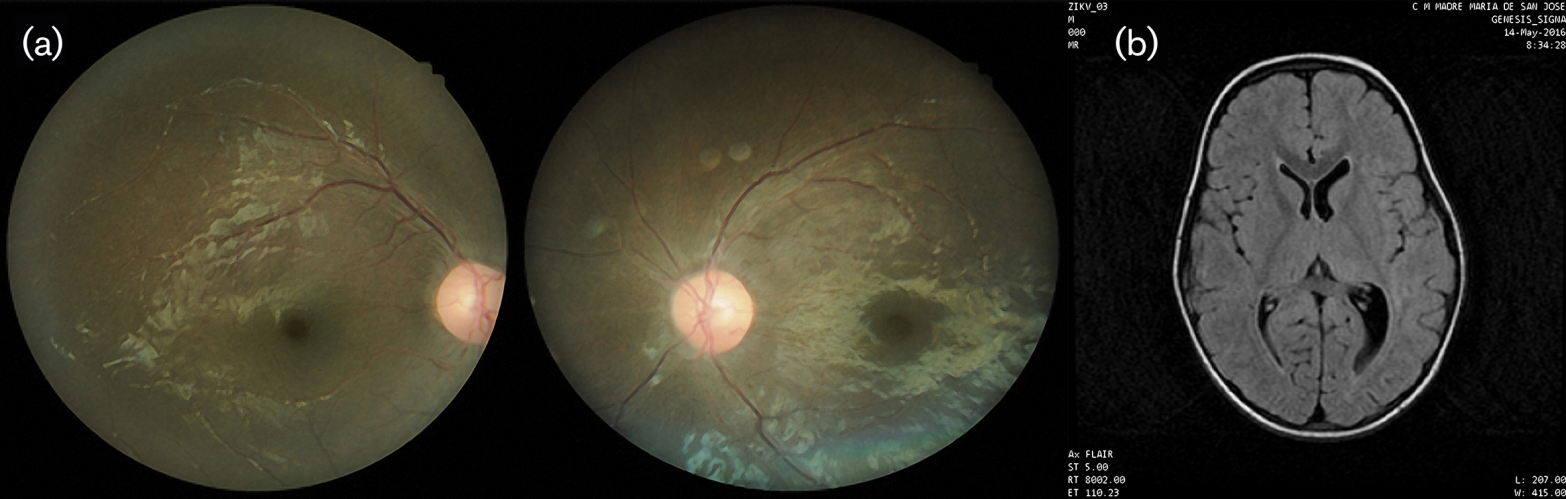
Patient described in Case 2. (a) Fundus examination revealed optic disc pallor and an edematous nerve fibre layer. Due to poor visual acuity, the patient was unable to perform visual field or optical coherence tomography (OCT) scans. (b) Magnetic resonance imaging (MRI) of the brain showing no abnormalities.
